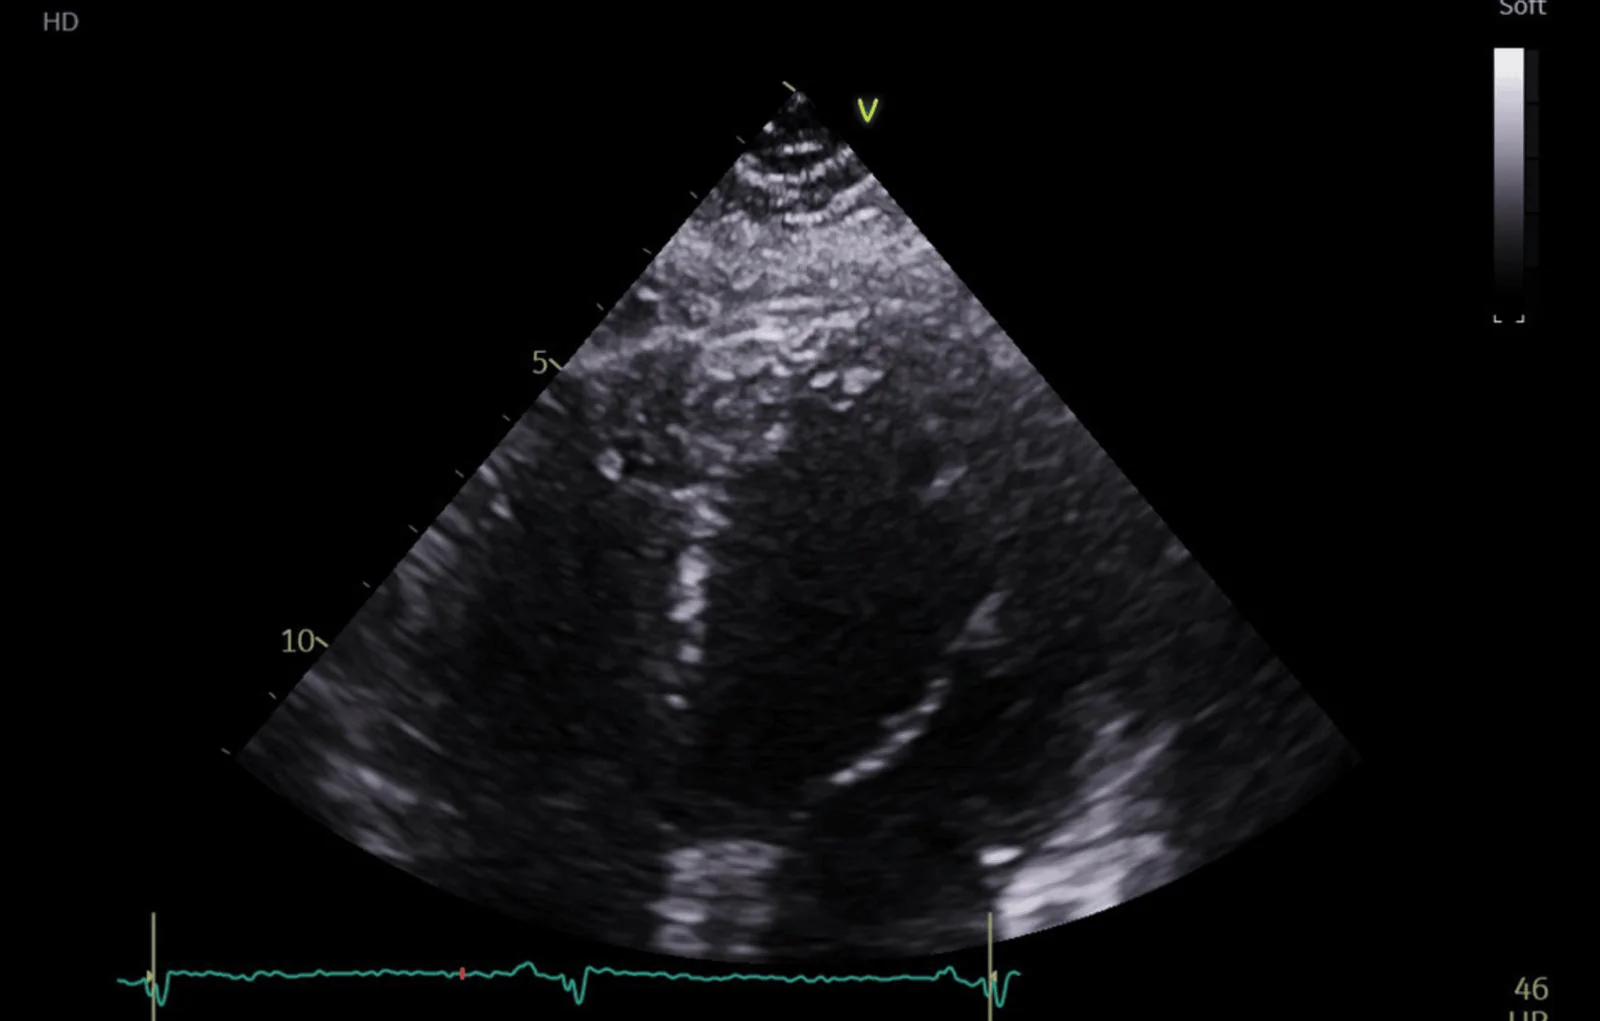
Transthoracic echocardiogram Transthoracic echocardiogram showing no regional wall motion defects or other abnormalities.
